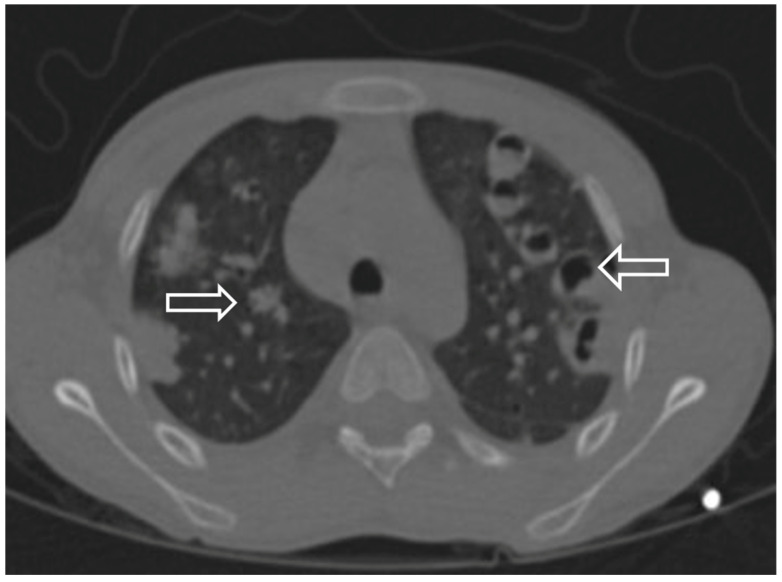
Contrast-enhanced chest computed tomography of a pediatric patient with septic pulmonary embolism showing nodular infiltrates and cavitations.
